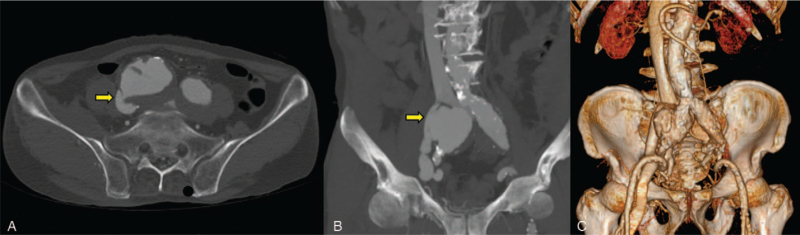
Contrast-enhanced computed tomography showing an isolated right common iliac artery aneurysm with evidence of an ilio-iliac arteriovenous fistula (arrow) in axial (A), sagittal (B), and 3D reconstruction (C) views. The contrast medium within right common iliac vein and inferior vena cava is visible.
